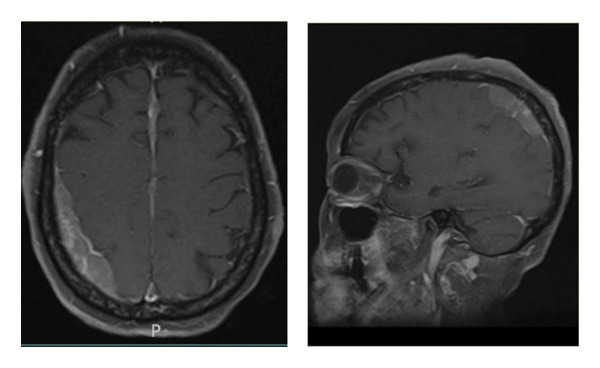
Axial and sagittal postcontrast T1‐weighted MRI brain obtained shortly after initial CT head concerning for subdural hematoma. MRI demonstrates dural thickening and enhancement along the bilateral cerebral convexities and interhemispheric falx, chronic microvascular ischemic changes, and chronic left basal ganglia infarction. There is stable abnormal decreased T1 marrow signal with heterogeneous enhancement, findings highly suggestive of an infiltrative neoplastic process rather than subdural hematoma.
